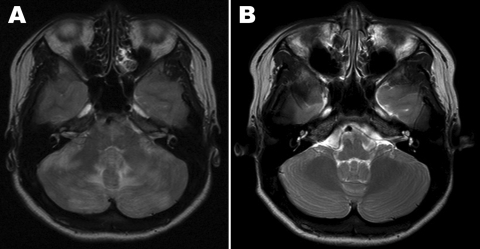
Magnetic resonance imaging (MRI) scans of case-patient’s brain. A) MRI at hospital admission shows ill-defined T2 changes in both cerebellar hemispheres, periventricular white matter, and the pons. B) MRI of the brain 1 month later, showing nearly complete disappearance of the changes observed at admission.
